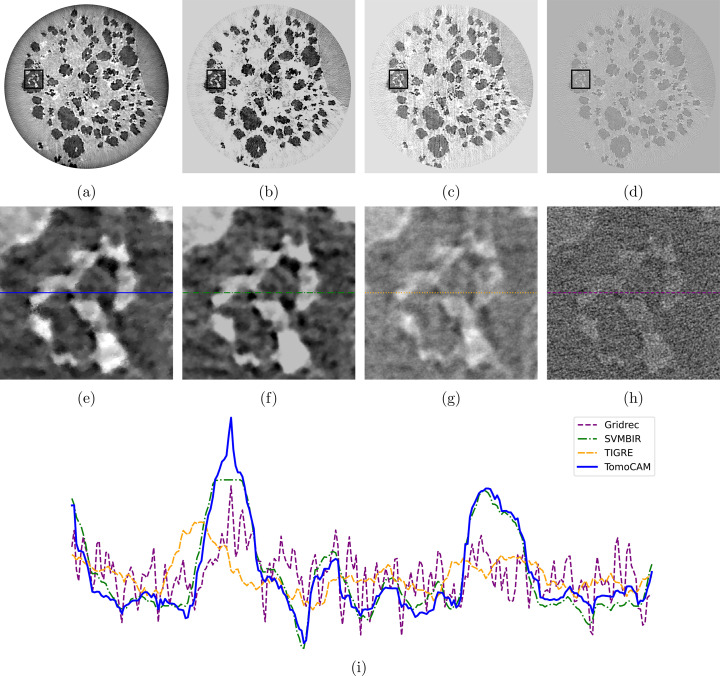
Reconstructions for Tomobank dataset ID 86, nano-CT data with sparse projection angles using 202 projections, with (*a*) *tomoCAM*, (*b*) *SVMBIR*, (*c*) *TIGRE* (IRN-TV-CGLS) and (*d*) gridrec. Panels (*e*), (*f*), (*g*) and (*h*) are zoomed-in regions of interest represented by the boxes in (*a*), (*b*), (*c*) and (*d*), respectively. Panel (*i*) displays the line profiles for (*d*), (*e*), (*f*) and (*h*). A circular mask was applied to all the reconstructions. While *tomoCAM* and *SVMBIR* both do an excellent job at suppressing the noise when compared with gridrec, *tomoCAM* is approximately 15× faster. Also, *tomoCAM* is 22× faster than *TIGRE* (IRN-TV-CGLS).
